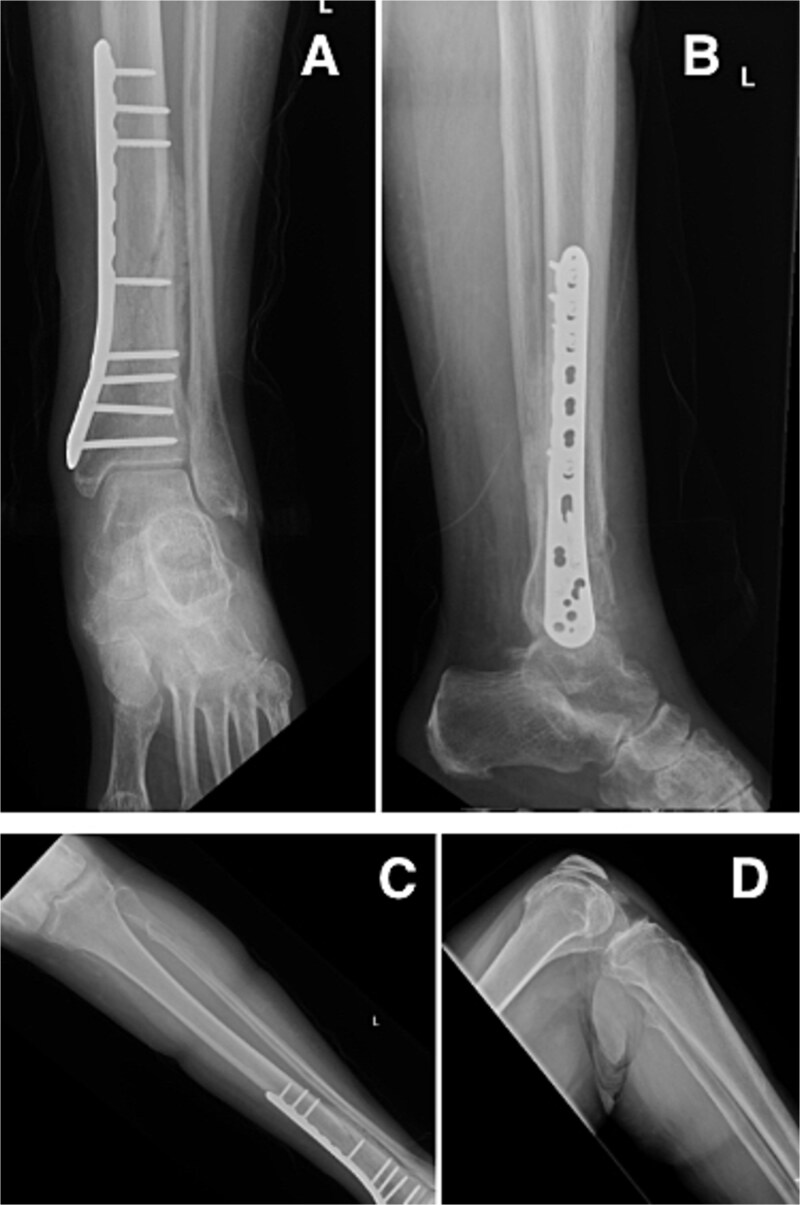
Radiographs at 3-month follow-up demonstrating healing of the left distal tibial spiral fracture and associated proximal fibular fracture. (A) Anteroposterior and (B) lateral views of the distal tibia show maintained alignment and progressive osseous union. (C) Anteroposterior and (D) lateral views of the proximal fibula demonstrate interval fracture healing.
